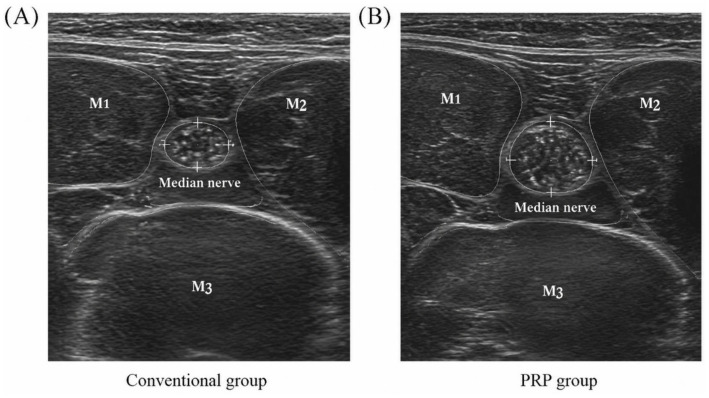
Ultrasound measurement of nerve cross-sectional diameter and area. (A) Representative sonographic image from the conventional group showing the median nerve at the injury site; (B) representative sonographic image from the PRP group showing the median nerve at the injury site. PRP, platelet-rich plasma.
